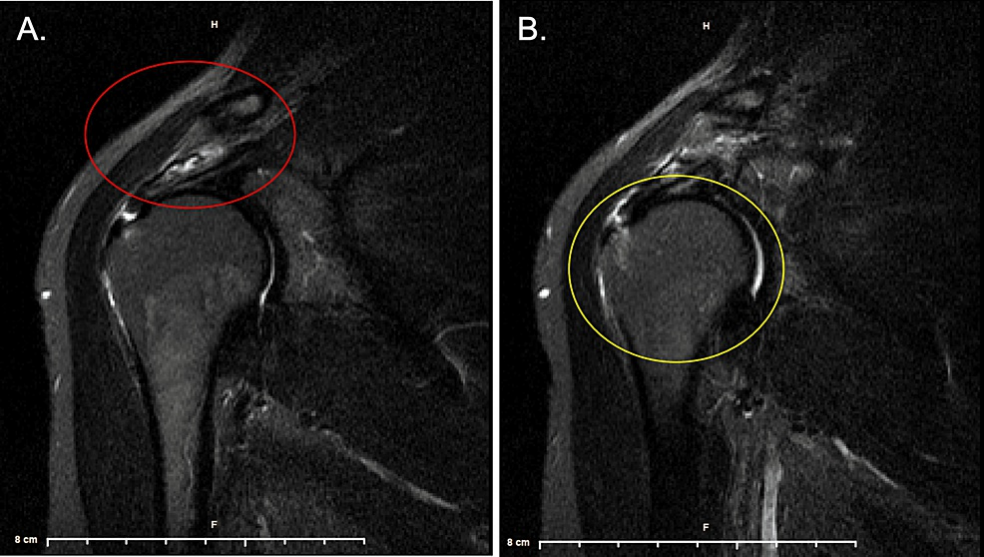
Magnetic resonance imaging of the right shoulder in coronal view with two millimeter partial tear in the distal supraspinatus tendon (A: red circle) along with subacromial-subdeltoid fluid in space (B: yellow circle).
